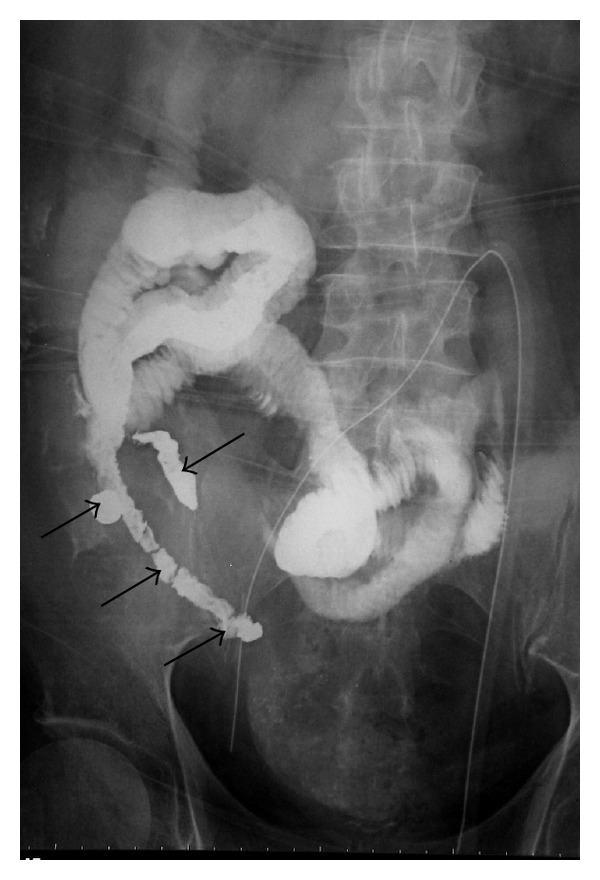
Supine abdominal radiograph. There is minimal visible bowel gas. Several loops of the small bowel are visible due to the presence of residual barium from a swallow study three days before. Segments in the right lower quadrant are particularly abnormal with the barium forming a dense cast-like appearance (arrows).
